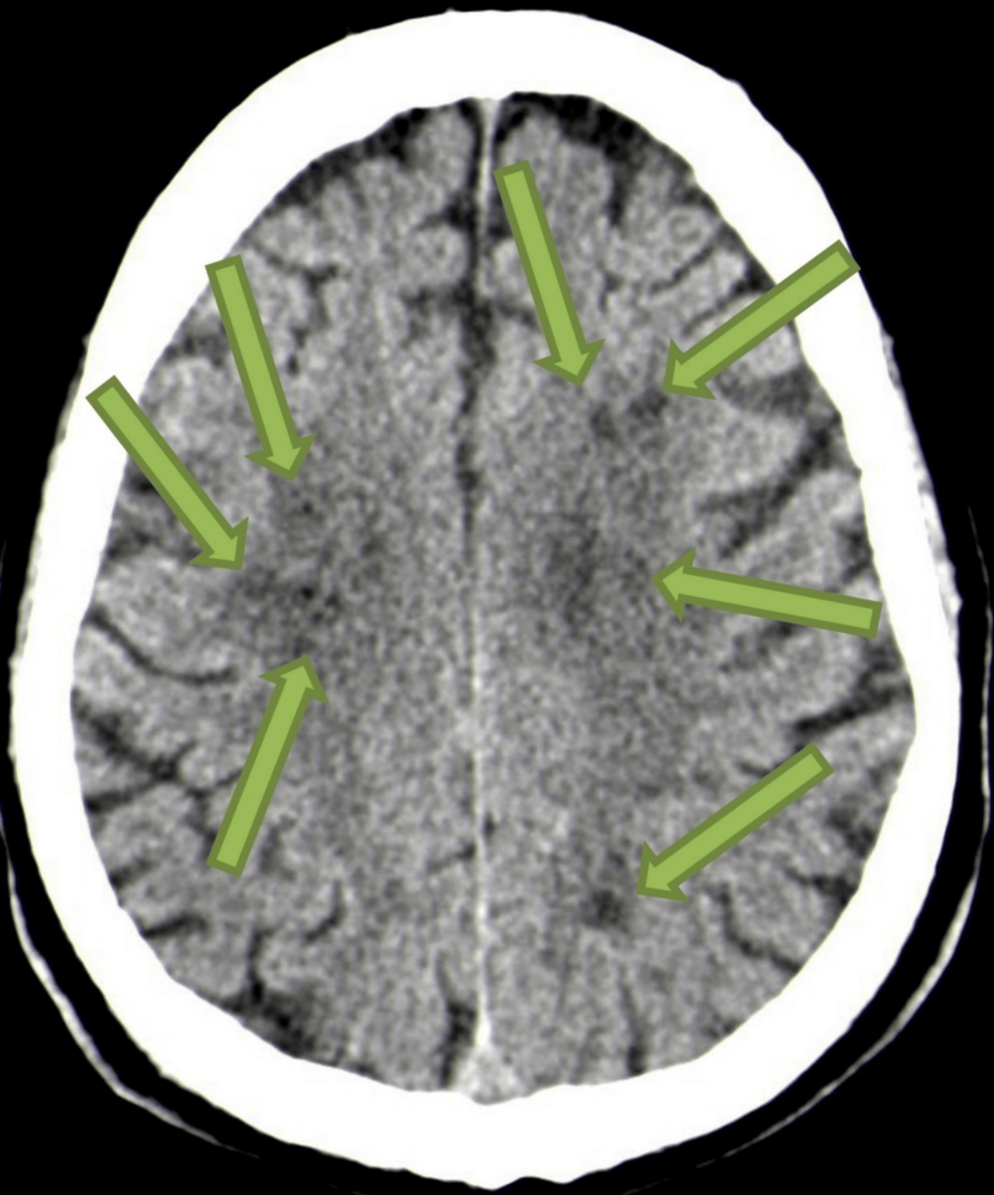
Cranial CT (axial plane): the green arrow indicates small focal lesions in the centrum semiovale.
